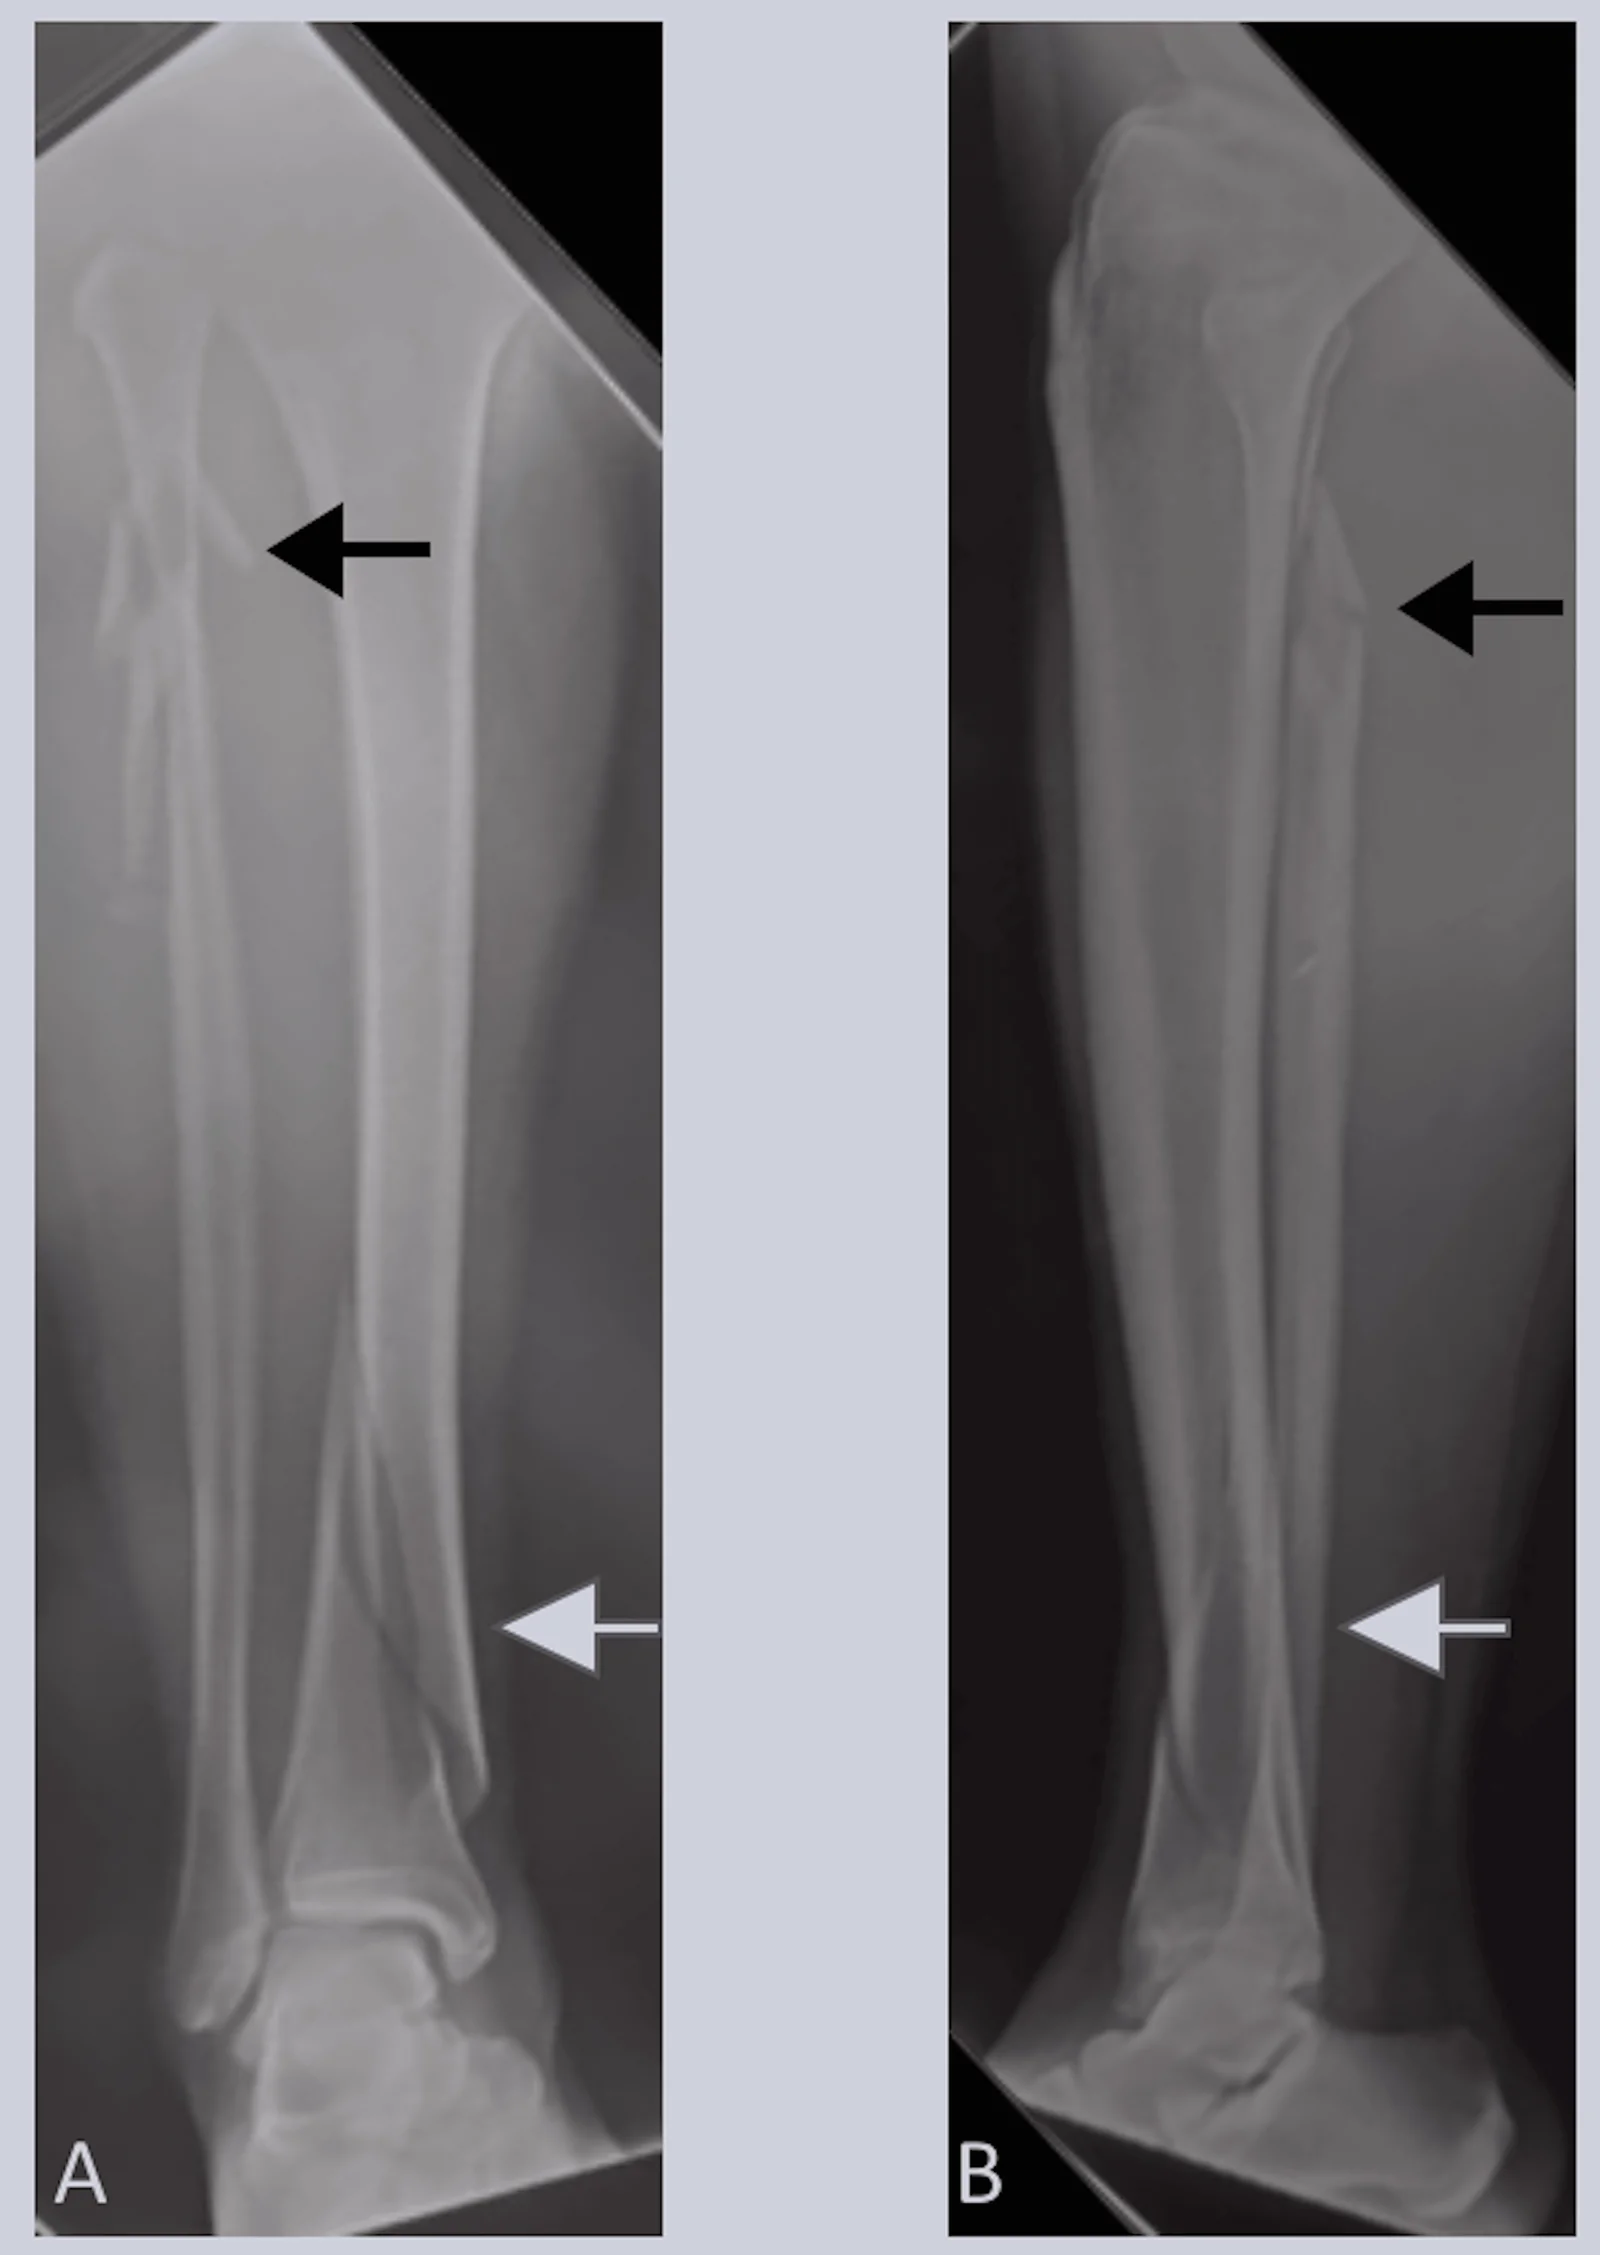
(A) Anteroposterior radiograph of the right tibia and fibula showing a distal tibia spiral fracture (white arrow) and a comminuted proximal fibula fracture (black arrow); (B) lateral radiograph of the right tibia and fibula showing a distal tibia spiral fracture (white arrow) and a comminuted proximal fibula fracture (black arrow).
